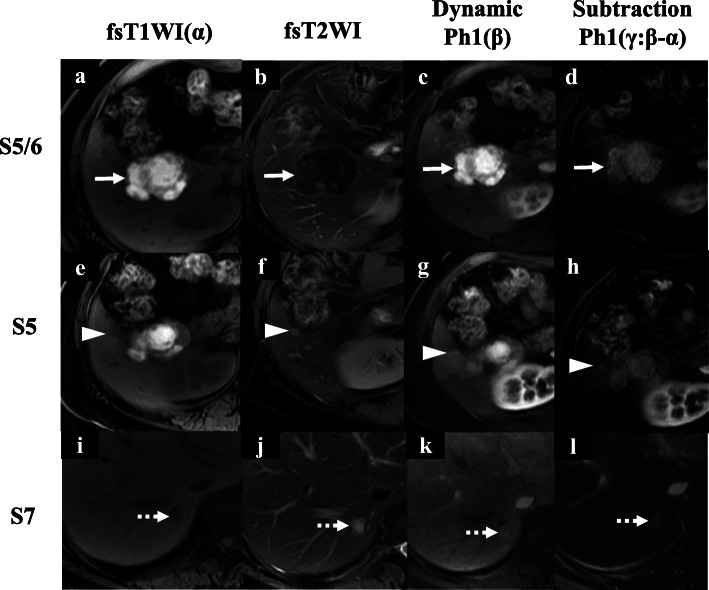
Gadoxetic acid-enhanced MRI (magnetic resonance imaging) findings. a–d S5/6 tumor showed very high-intensity regions on fat suppression T1-weighted imaging (fsT1WI), low intensity on fsT2WI, high intensity in dynamic Ph1, and strong enhancement in subtraction images (dynamic Ph1 – fsT1WI) (white arrow). e–l S5 and S7 tumors were visualized as low-intensity regions on fsT1WI, high intensity on fsT2WI, iso-intensity in dynamic Ph1, and weak enhancement in subtraction images (dynamic Ph1 – fsT1WI) (white arrowhead and dotted white arrow)
